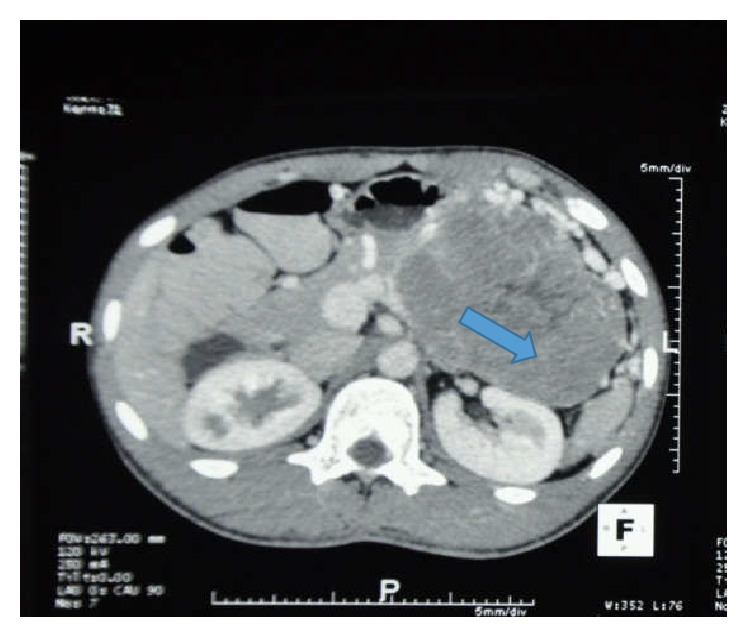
CT image demonstrating presence of a heterogeneous well-demarcated mass arising from the body and tail of the pancreas (marked with a blue arrow) and extending towards the splenic hilum.
